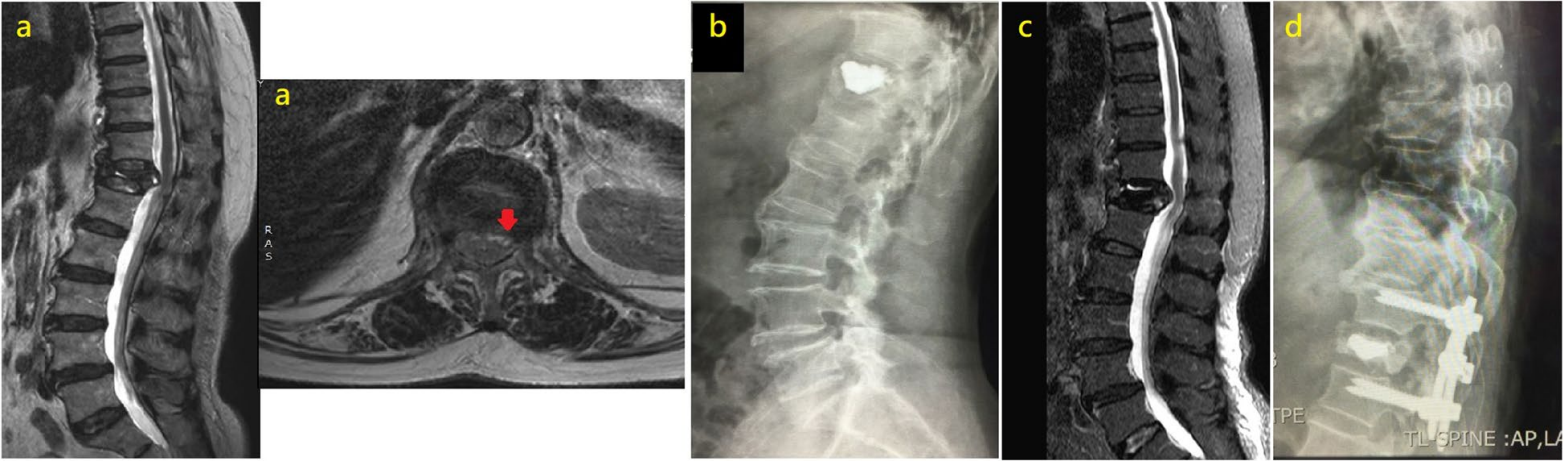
MRI showing T12 compression fracture with posterior vertebral rim disruption (red arrow) (a). T12 PKP was performed (b). Patient complained of right groin pain (L1 dermatome) 4 months after PKP, MRI proving spinal canal compression at T12 level (c). We performed T11-L1 level posterior decompression and instrumentation (d)
